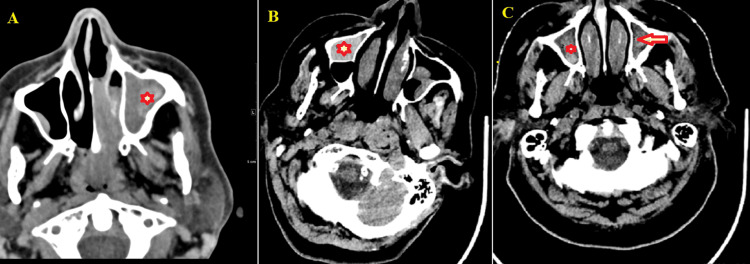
Non-enhanced computed tomography (CT) scans of the paranasal sinuses and the brain (A) Non-enhanced computed tomography (CT) scan of the paranasal sinuses with a soft tissue window demonstrates complete inhomogeneous opacification of the left maxillary sinus (asterisk). Within this sinus, the hyperdense material with an average density of 85 Hounsfield units (HU) is centrally located and surrounded by hypodense mucosa. This finding was pathologically proven to be allergic fungal sinusitis. (B) Non-enhanced CT scan of the brain demonstrates bilateral maxillary sinus retention cysts. The right maxillary sinus (asterisk) displays a hyper-attenuated retention cyst with an average density of 58 HU. (C) Non-enhanced CT scan of the brain reveals bilateral maxillary sinus mucosal thickening, with hyperdense materials exhibiting an average density of 37 HU in the left maxillary sinus (arrow) and 35 HU in the right maxillary sinus (asterisk). These findings are indicative of chronic sinusitis with inspissated secretion.
